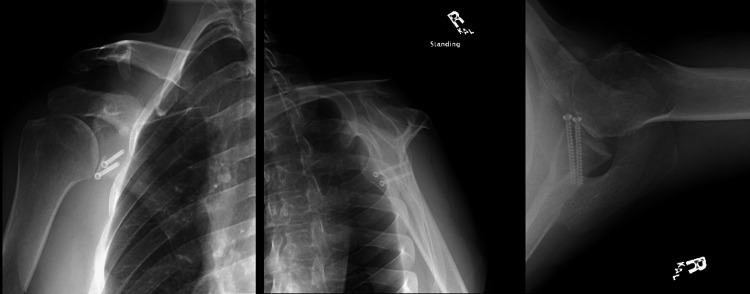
Radiographs of the right shoulder at three-month follow-up from case 1
